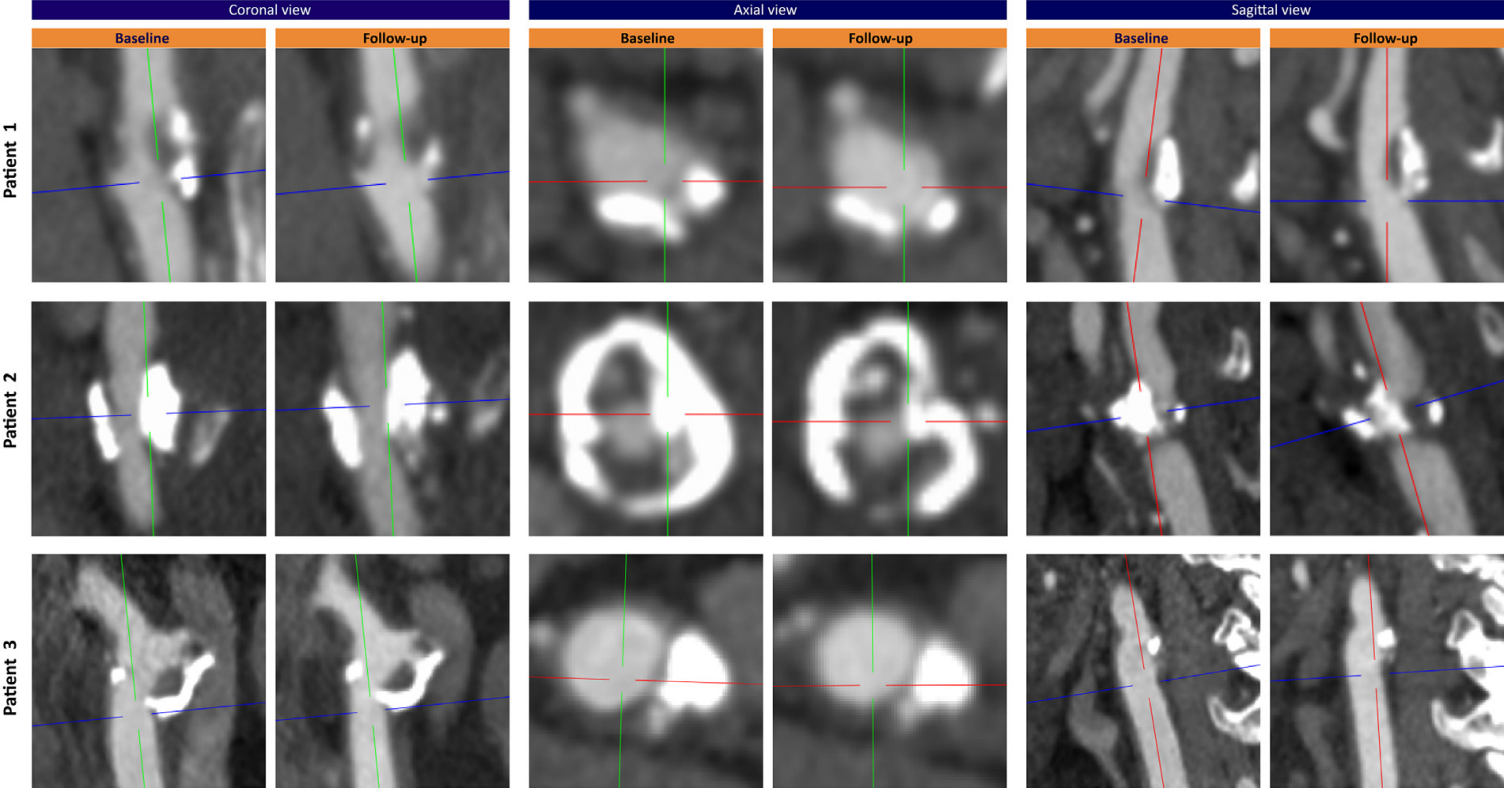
Baseline and follow-up CTA of patients with decreasing ECAC. CTA images in axial, sagittal, and coronal views at baseline and 2-year follow-up of patients with decrease in ECAC.
